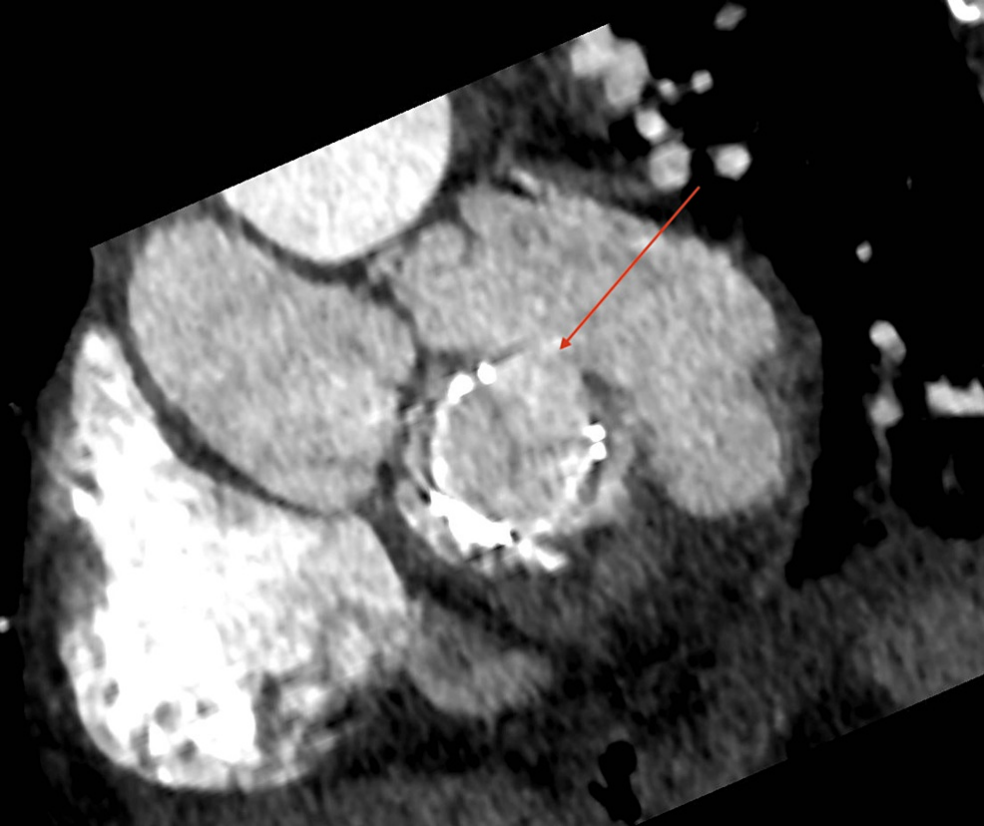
Cardiac computed tomography in the short-axis view. The red arrow shows the opening of the neck of the left ventricular pseudoaneurysm in relation to the mitral valve prosthesis.
